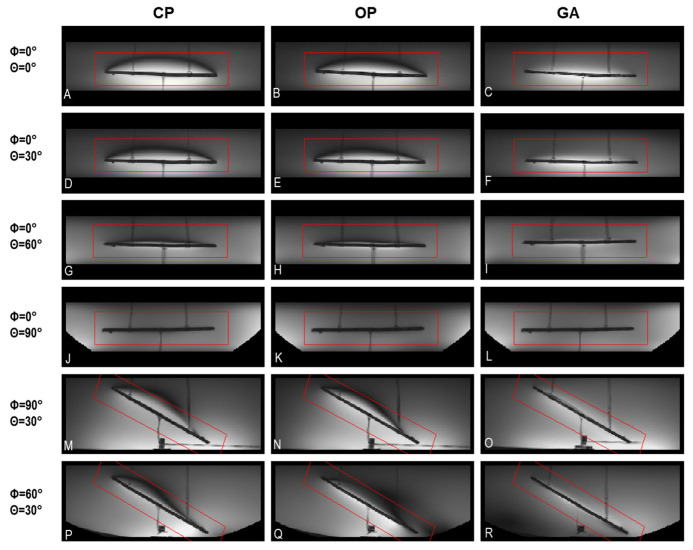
Minimum-intensity projections of worst-case $B_{1}^{+}$ artifacts obtained from 3D gradient-echo MRI in the vicinity of the implant using CP-, OP-, and GA-based transmission field shimming; (A–R) correspond to the excitation vector shown in Figure 7 for several implant orientations. The implant orientation is defined by using polar and azimuthal angles in spherical coordinates by considering the origin of the coordinate system on the center of the implant. The ROI under investigation is indicated in red.
